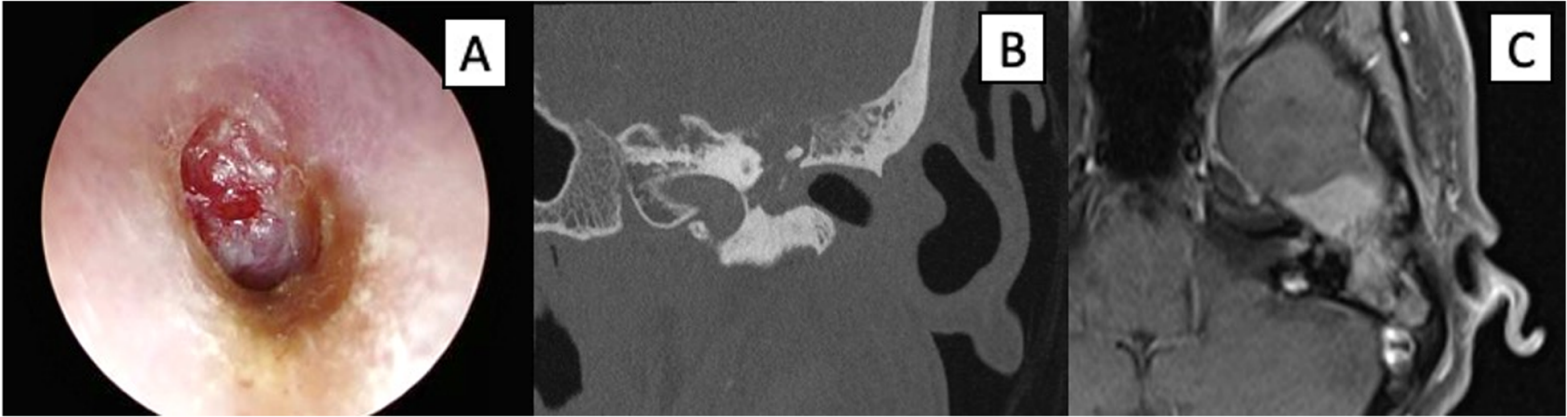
Pre-treatment clinical images. a Endoscopic view of left ear canal and middle ear mass replacing the tympanic membrane. b Coronal computed tomographic image of left temporal bone showing erosion of the tegmen tympani (note the permeative bony changes), malleus and facial nerve canal. c Axial magnetic resonance imaging view of the left middle ear mass involving the middle cranial fossa and extending into the internal auditory canal
